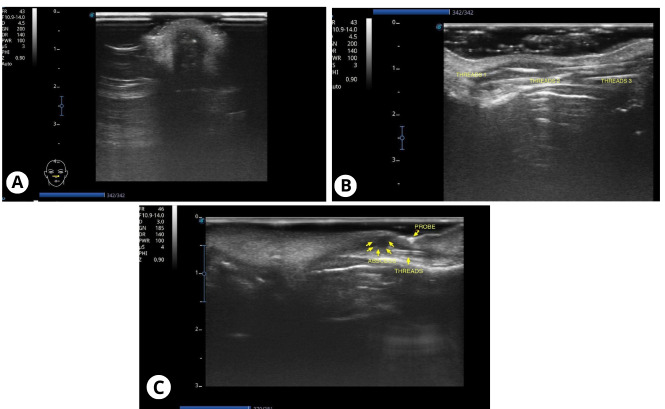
Point of care ultrasound (POCUS) images of the nose. A: A diffuse anechoic area is observed at the tip of the nose, associated with a surrounding hyperechoic region concerning for abscess. B: Three elongated hyperechoic lines are seen along the long axis of the nasal dorsum, inserted in the subcutaneous tissue, consistent with polydioxanone (PDO) threads and surrounded by diffuse anechoic areas (yellow arrows) extending from threads. C: PDO threads in the nose with abscess.
